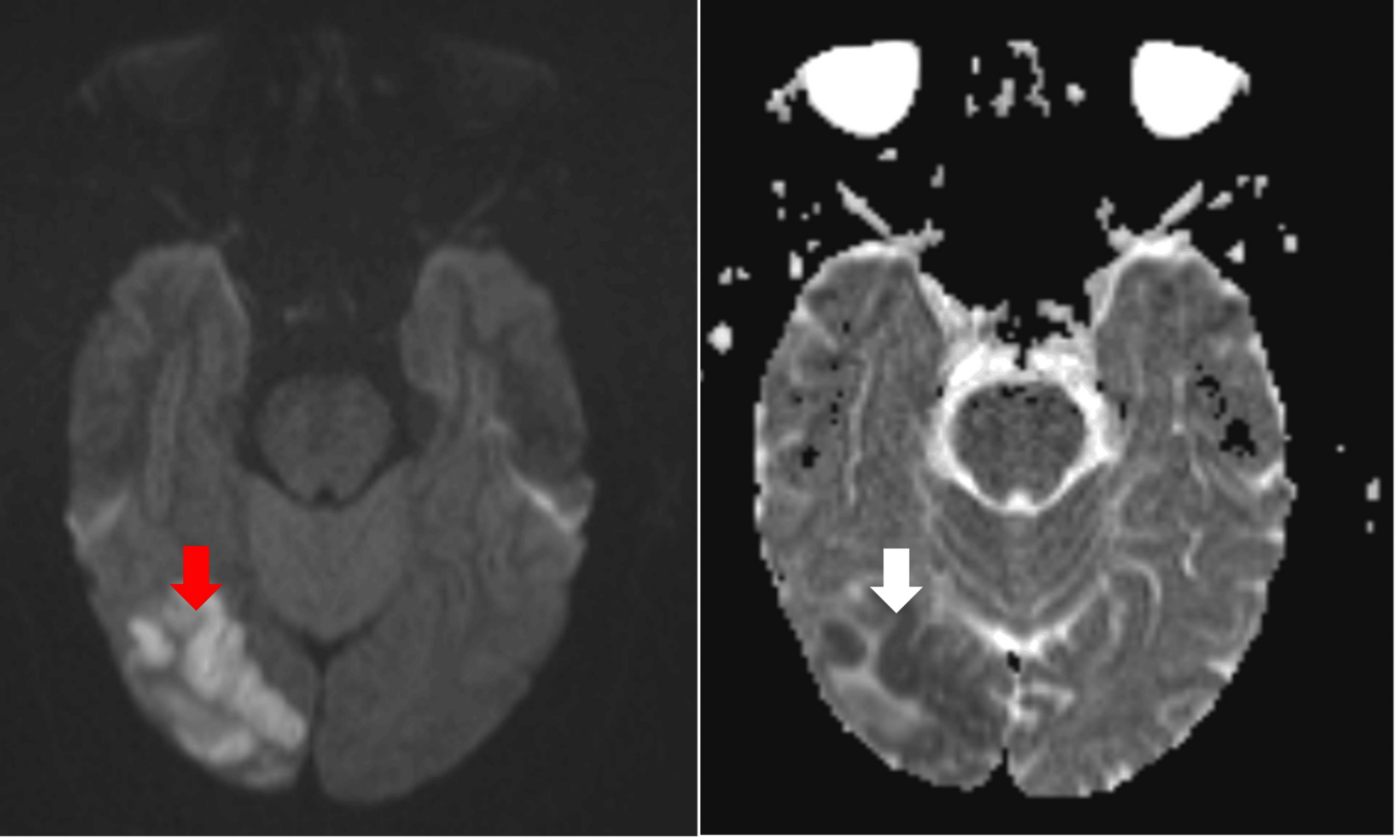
MRI brain showing restricted diffusion in the right occipital lobe on diffusion-weighted imaging (DWI) (red arrow) with corresponding hypointensity on apparent diffusion coefficient (ADC) (white arrow)
